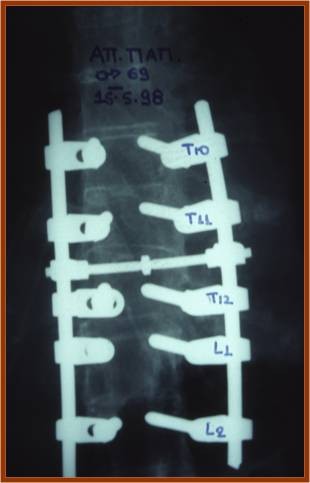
Anteroposterior postoperative radiograph with a posterior instrumentation system.
